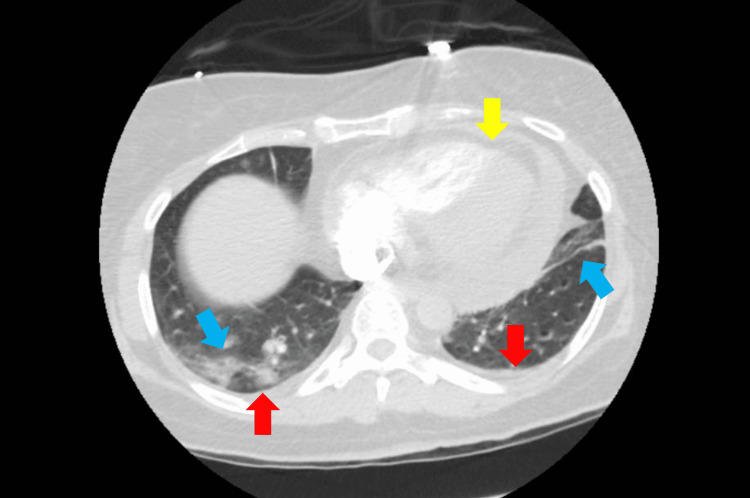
CT scan of the chest with contrast showed moderate pericardial effusion (yellow arrow), bilateral patchy infiltrates (blue arrow), and trace bilateral pleural effusion (red arrow).
